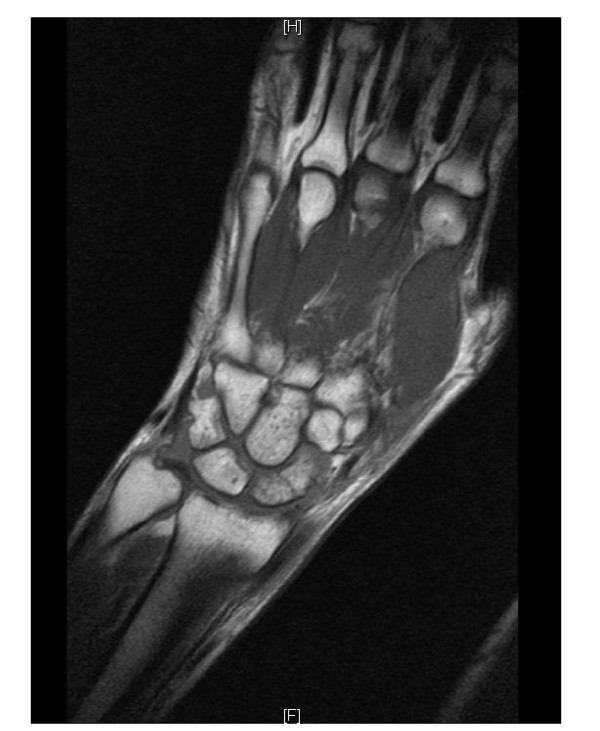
Magnetic resonance imaging (patient D) of a waist fracture of the scaphoid.
